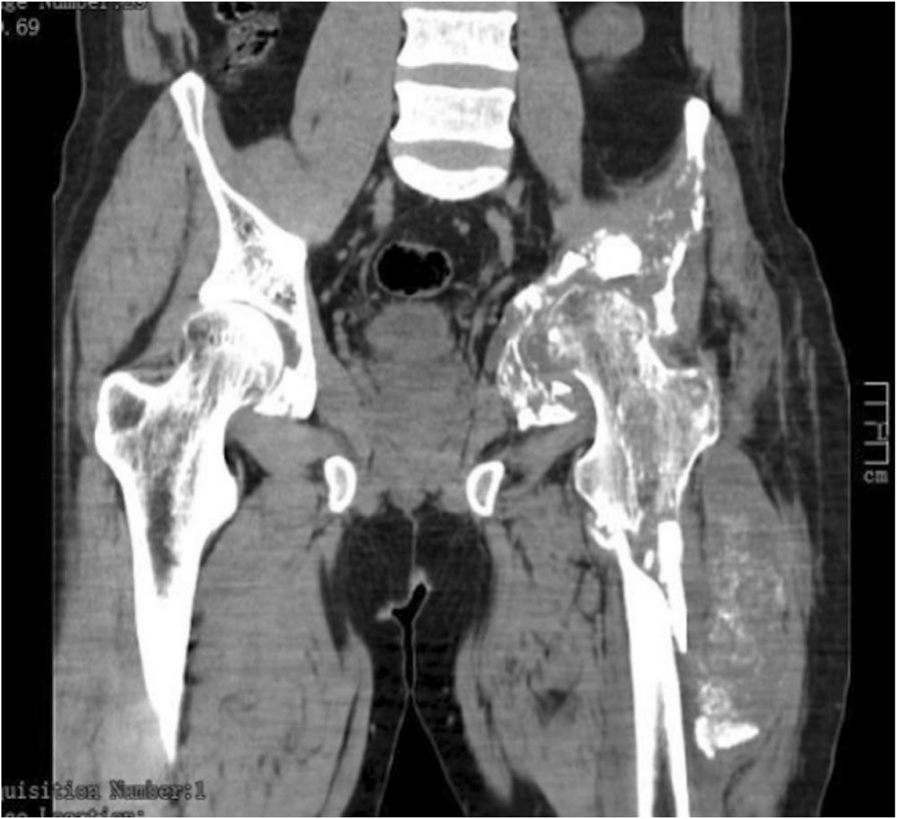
The CT coronal plane showed that the soft tissue around the upper left side of the femur was swollen, showing cystic changes, with irregular calcification in the cyst
